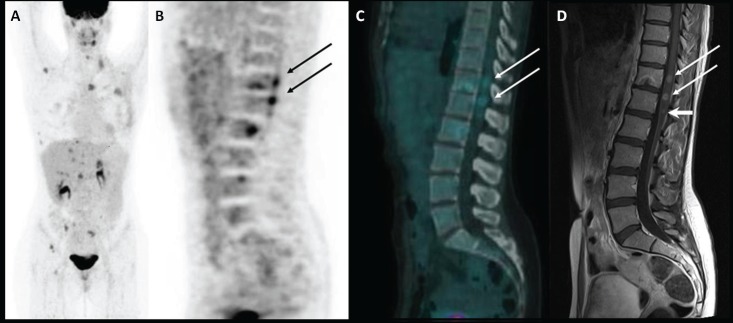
Maximum intensity projection image of 18F-FDG PET/CT scan (A); PET, CT, and MRI sagittal images of spinal cord metastases (B, C, and D respectively).
